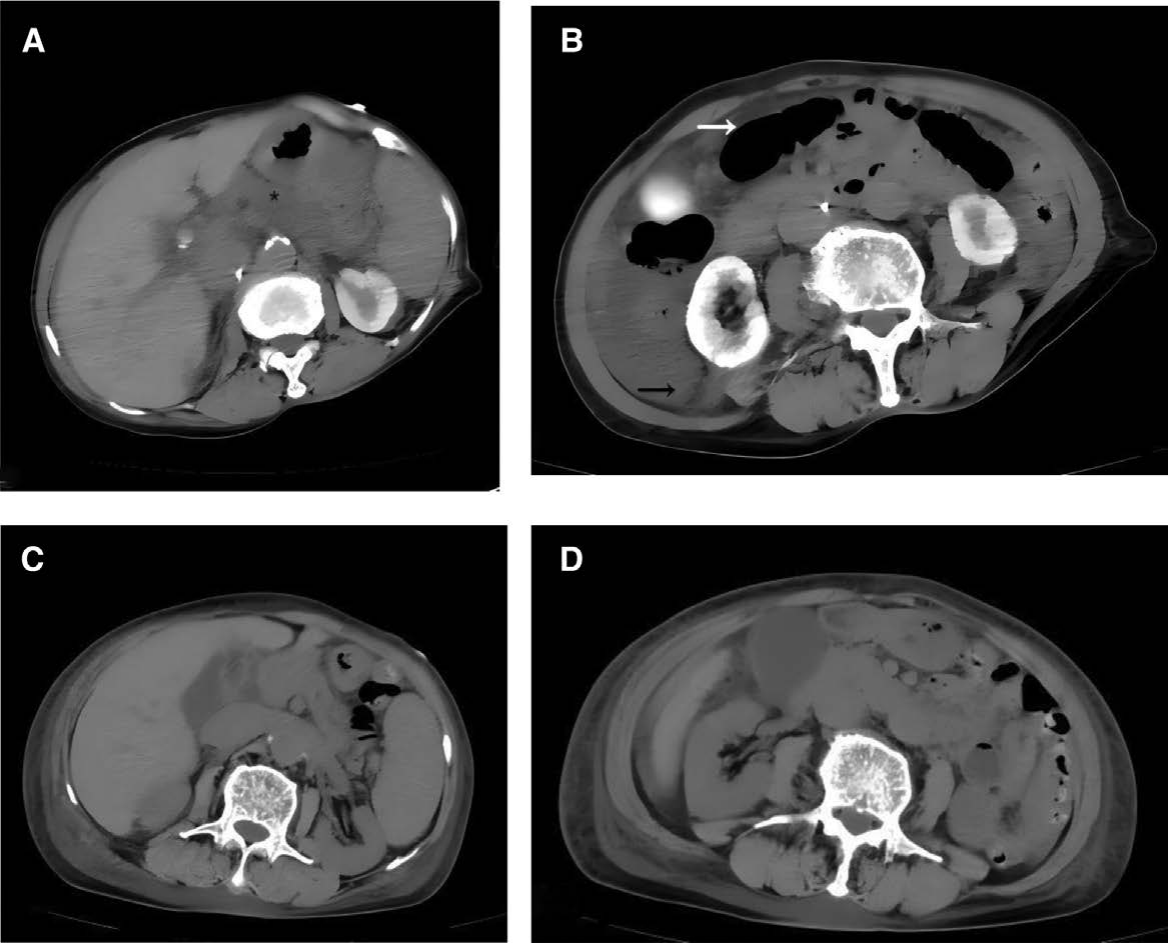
(A,B) Abdominal CT, * unclear outline of pancreatic body, → white arrow intestinal gas accumulation, black arrow ascites. (C,D) Abdominal CT, resolution of retroperitoneal effusions. CT = computed tomography.
